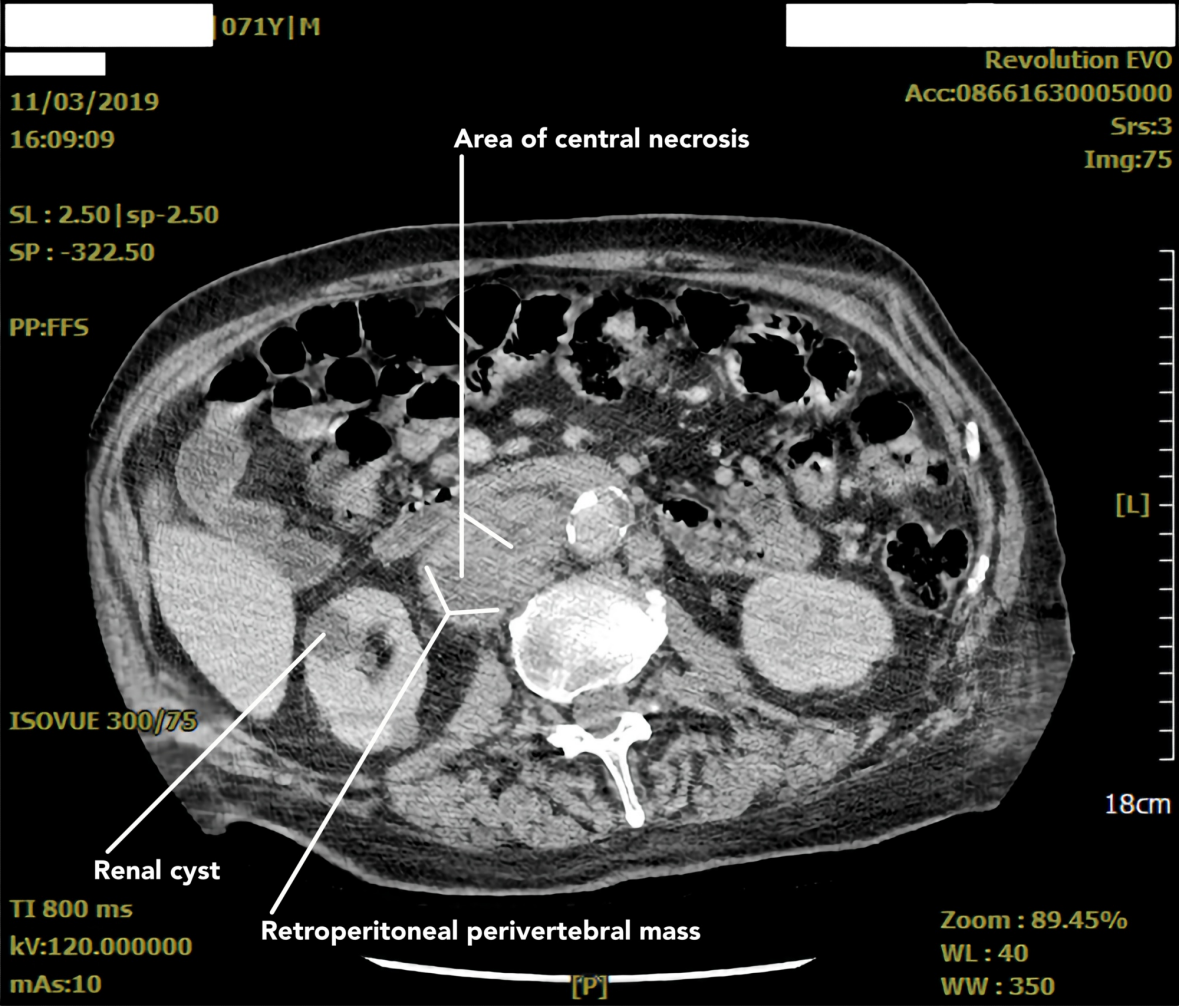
Abdominopelvic CT scan with contrast shows retroperitoneal masses including a heterogenous mass adjacent to the aorta to the right of the midline at the level of the kidneys measuring 11.9 cm in the largest dimension.
